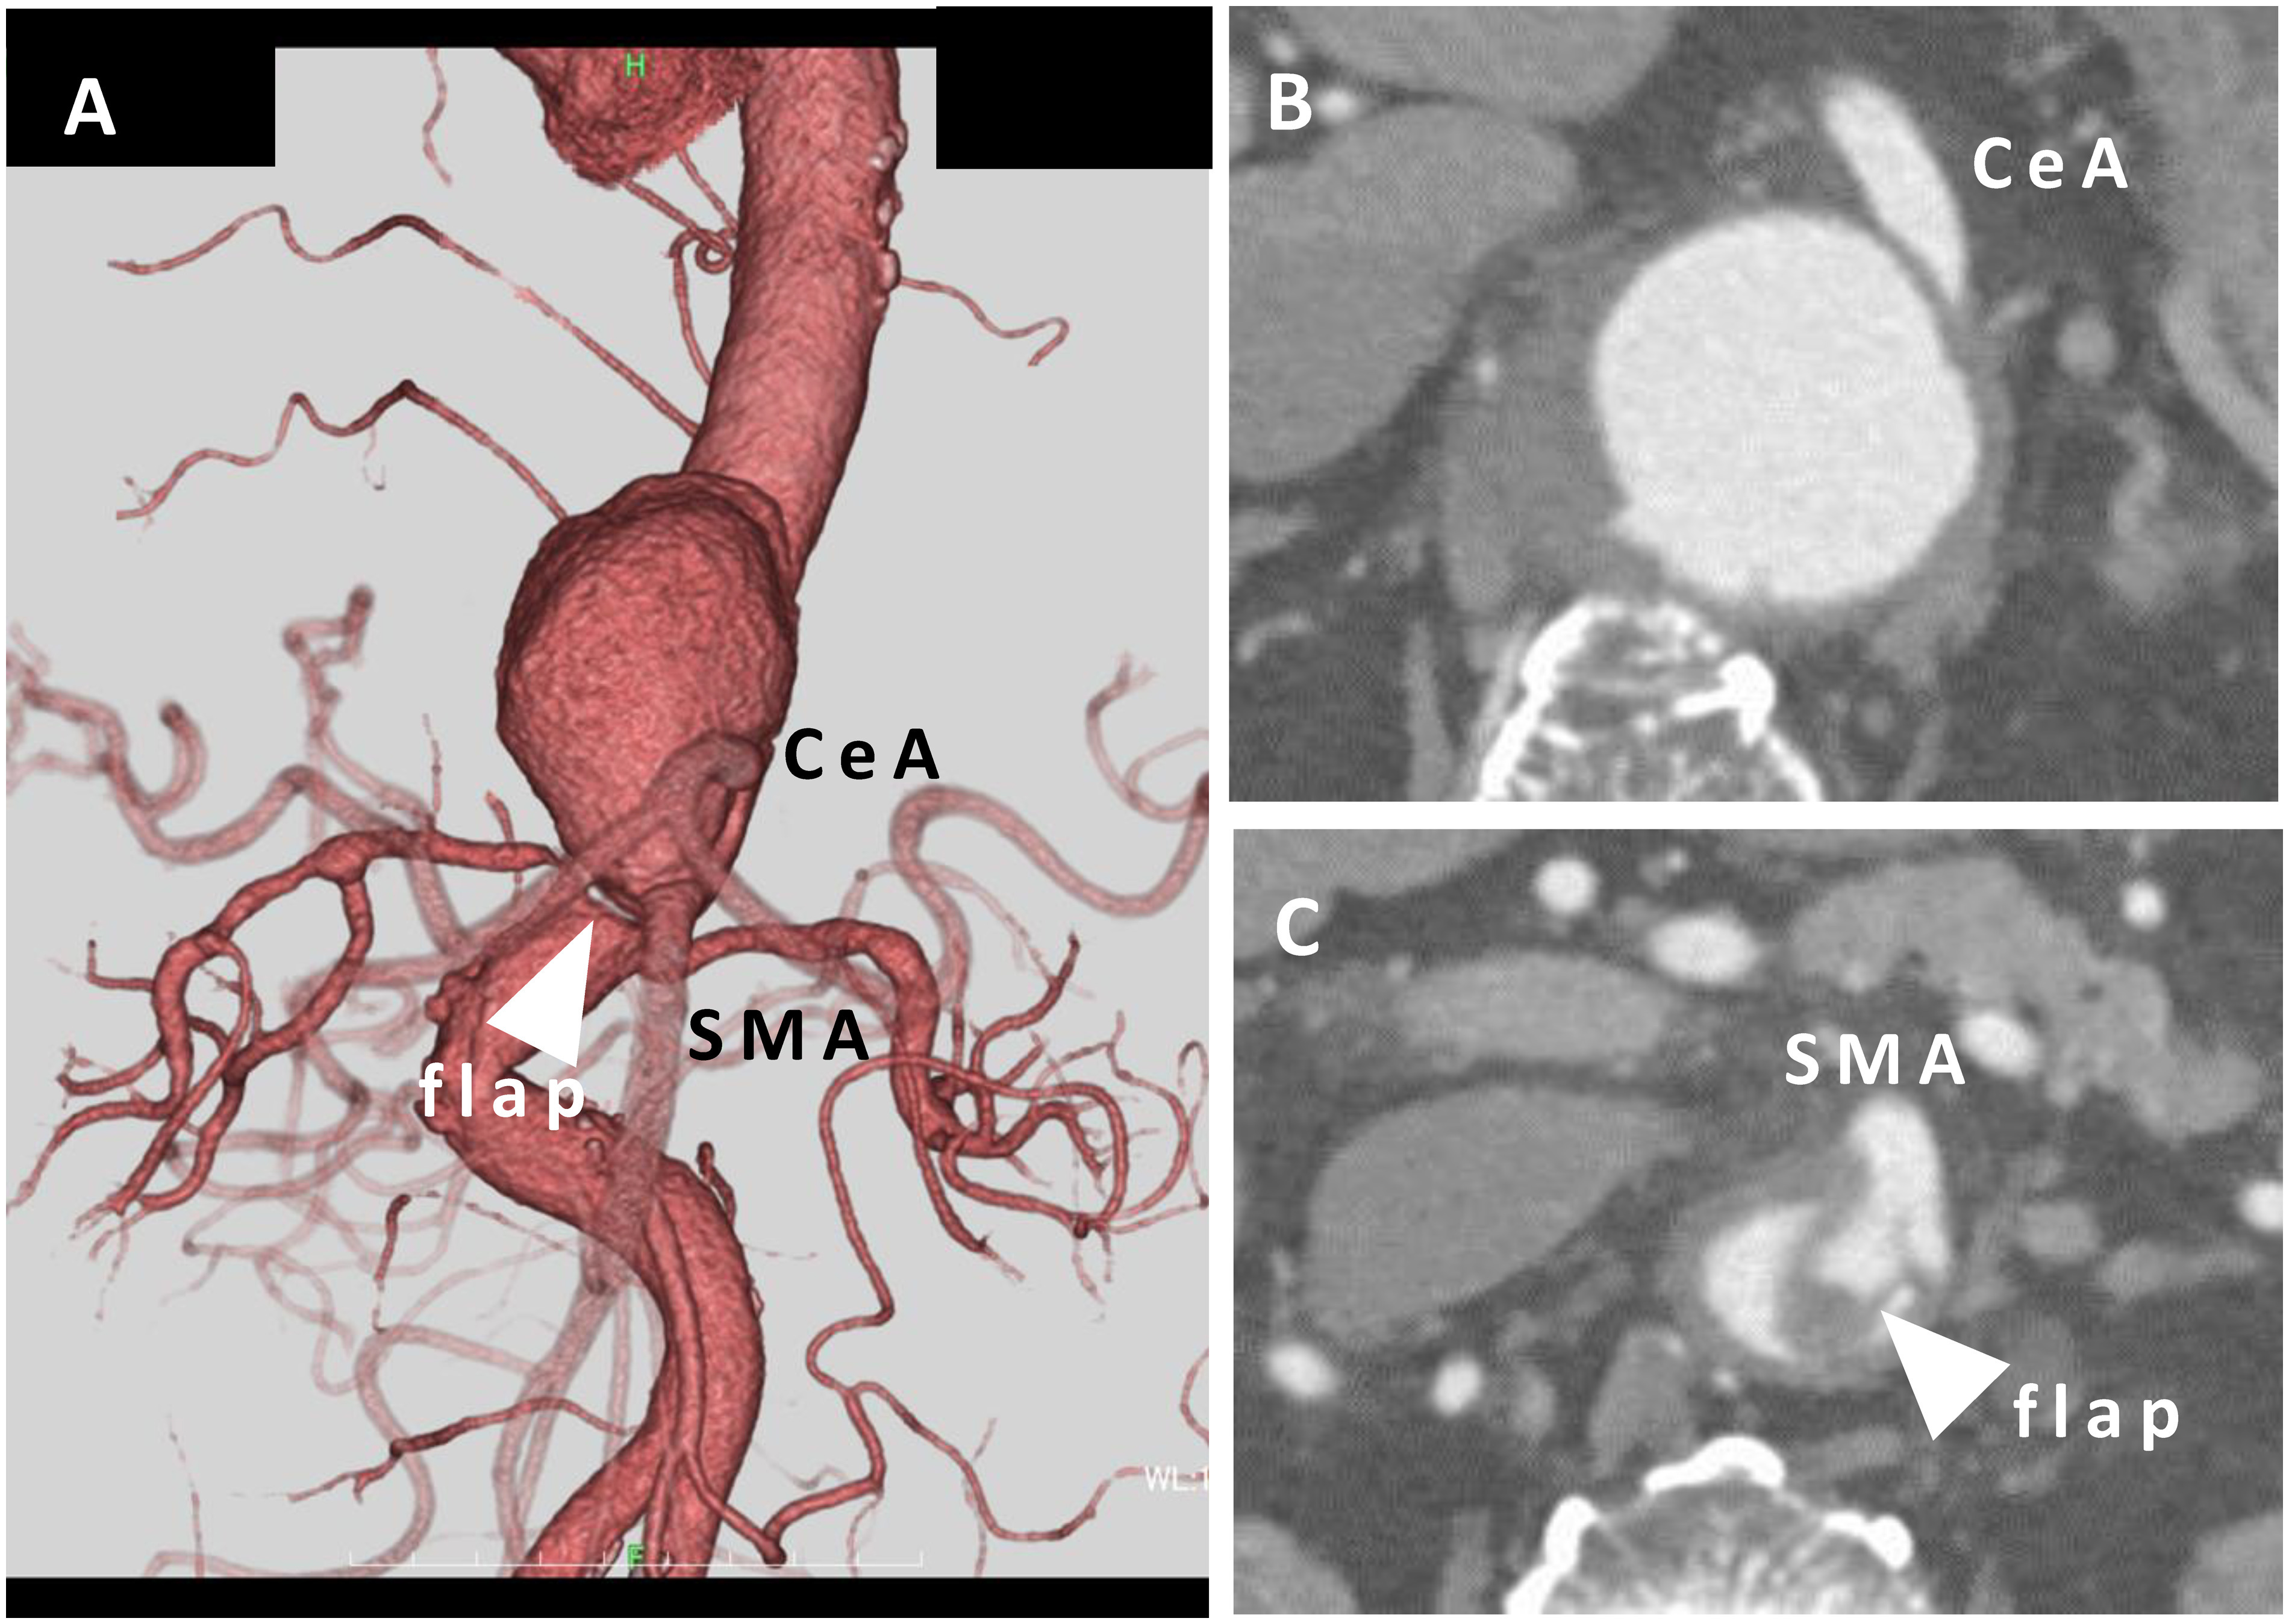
Fig. 1 Contrast-enhanced CT scan. (A) 3 dimension CT angiography. There existed an intimal flap due to localized dissection between the SMA and bilateral RAs. (B) Celiac artery branched from the 53 mm thoracoabdominal aortic aneurysm. (C) Dissection flap was observed at a level of an orifice of the superior mesenteric artery. An entry tear was not detected.
